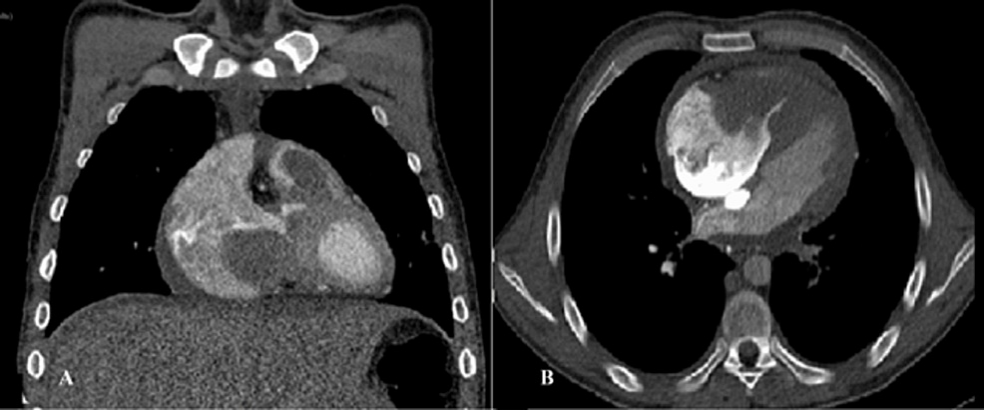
Cardiac multislice computed tomography showing a large mass occupying the right heart - (A) coronal view and (B) axial view.
